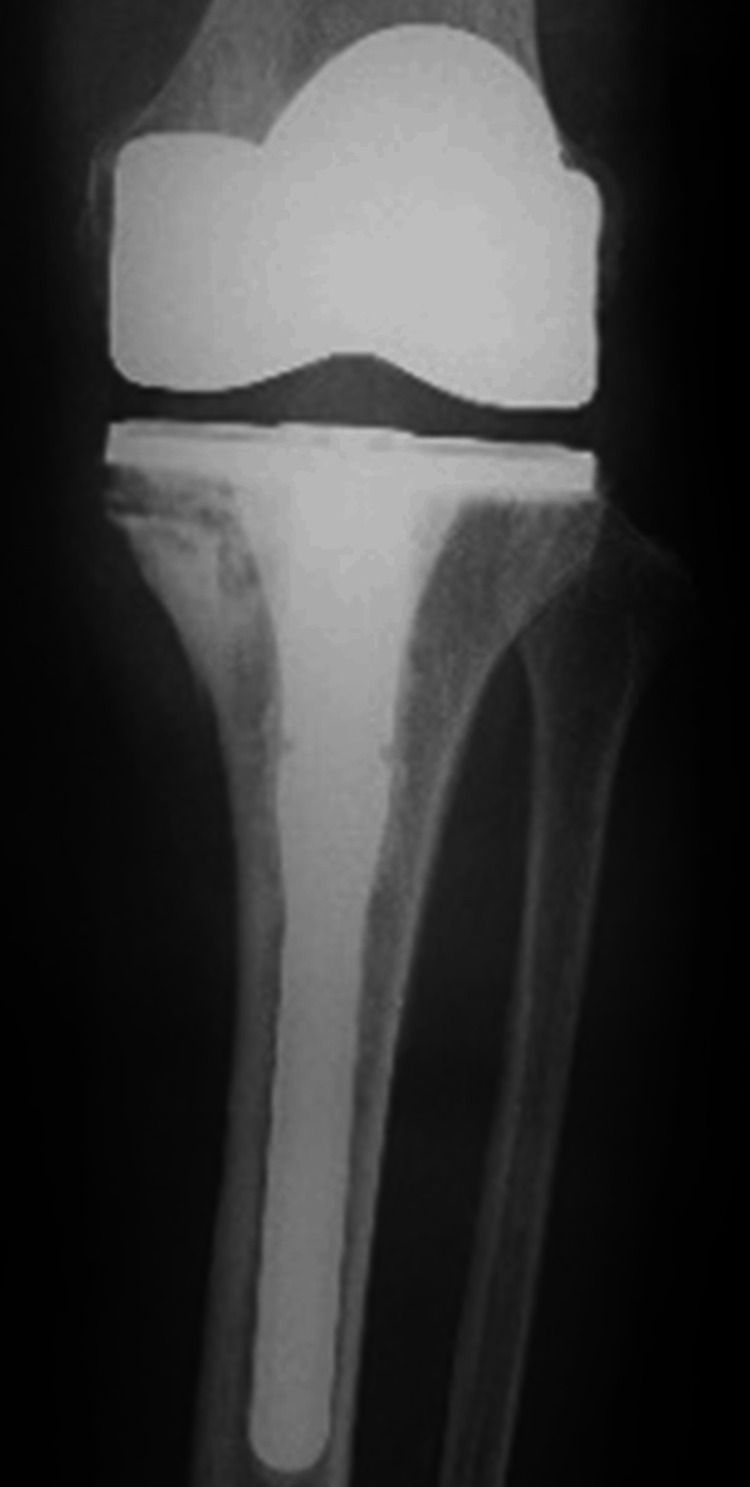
Radiography after conversion to TKA (AP view) TKA: total knee arthroplasty, AP: anteroposterior.
